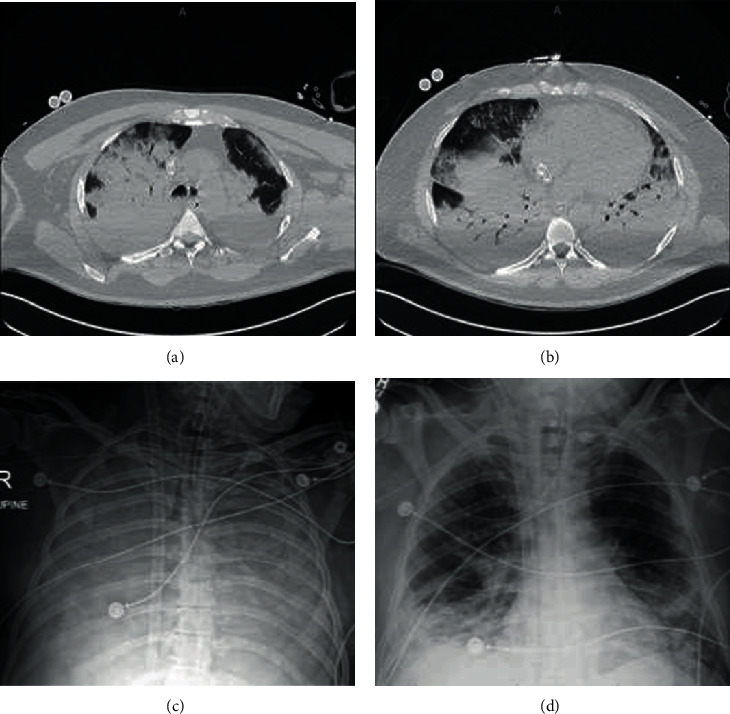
(a, b) Computed tomography of the chest immediately following ECMO cannulation (HD1) demonstrating dense consolidative opacities bilaterally. (c) Chest X-ray from HD8 demonstrating complete opacification of lungs bilaterally. (d) Chest X-ray from HD12 just prior to ECMO decannulation showing marked improvement aeration of bilateral lungs.
